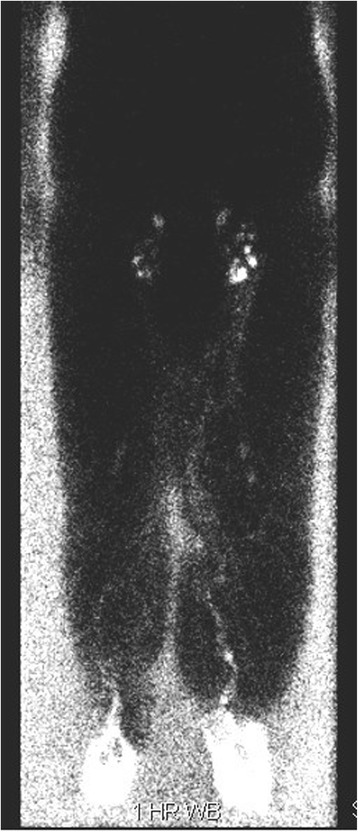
Bilateral lower extremity lymphoscintigram of patient with bilateral congenital lower extremity lymphedema tarda, demonstrating lymphatic webbing, dermal backflow and secondary nodal drainage basins. Note the presence of groin nodes on this 6 h post-injection image
